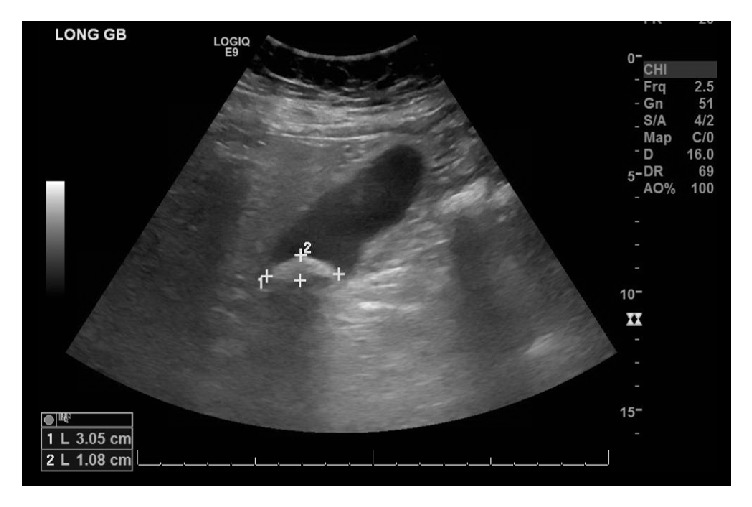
Preoperative ultrasound shows cholelithiasis.
